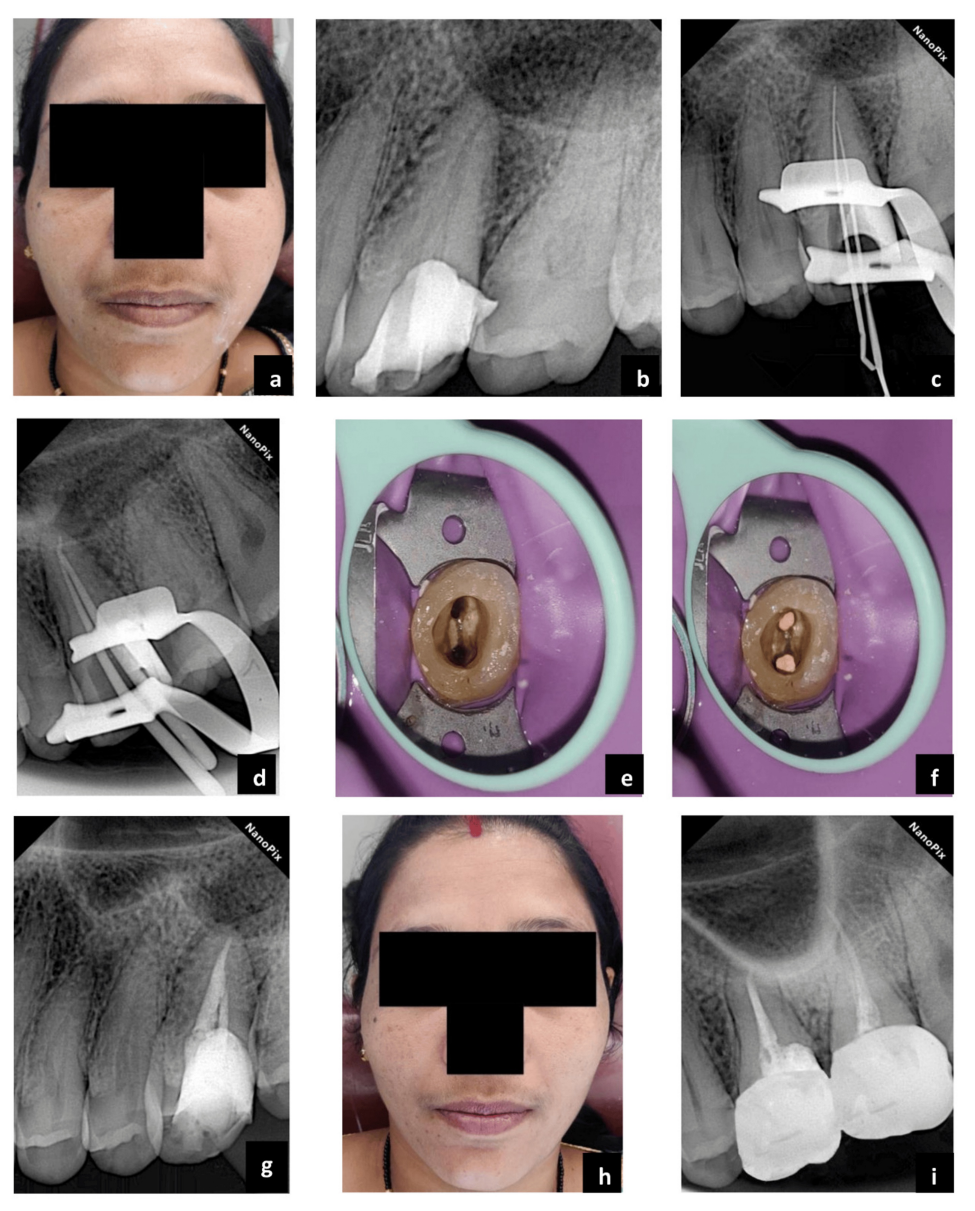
Endodontic treatment with #26 legend. (a) Preoperative extraoral photograph - facial asymmetry in the left cheek region, (b) preoperative radiograph of tooth #26, (c) working length radiograph, (d) master cone radiograph, (e) access opening clinical image, (f) postobturation clinical image, (g) postoperative radiograph, (h) postoperative extraoral photograph, and (i) 18 months follow-up image.
